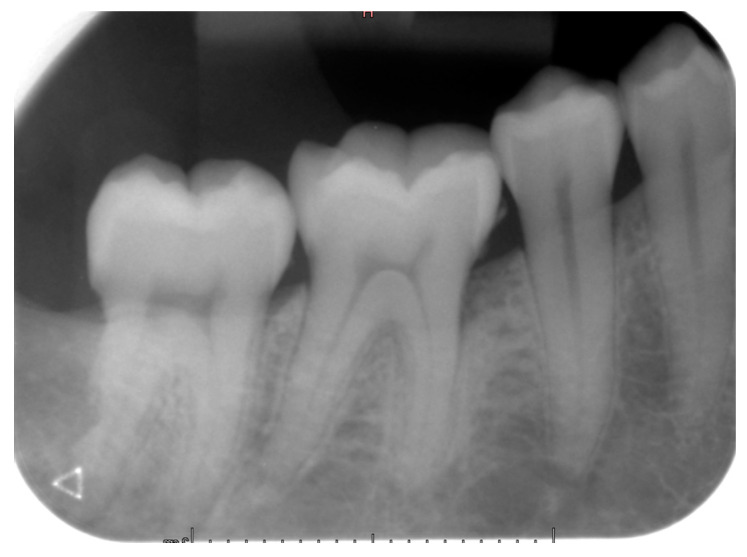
Intraoral X-ray of case #2 at one-year follow-up exam.
